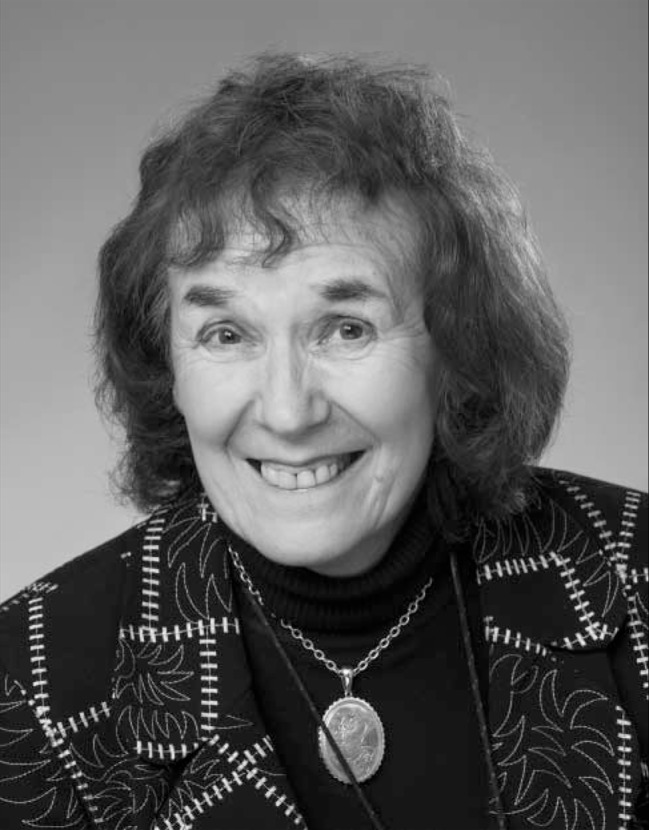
As a southerner and medical psychotherapist, it was with some trepidation that I ‘Ubered’ to Professor Eve Johnstone's house in Edinburgh, which boasts over a hundred roses in her immaculate garden. After all, I was going to meet the woman whose research work had wrenched the pendulum radically in the direction of biological psychiatry, with her landmark *Lancet* paper^1^ showing anatomical differences on CT scans between the brains of those with and without schizophrenia. I feared that she ate psychotherapists for breakfast, although she had sounded kind and friendly on the telephone. My anxiety was misplaced, since the person who emerged was a woman of fierce intelligence, with rigorous, idealistic, uncompromising and wholly admirable moral standards; compassionate and committed to patients and science, without any personal vanity, unless you include intellectual certainty.
